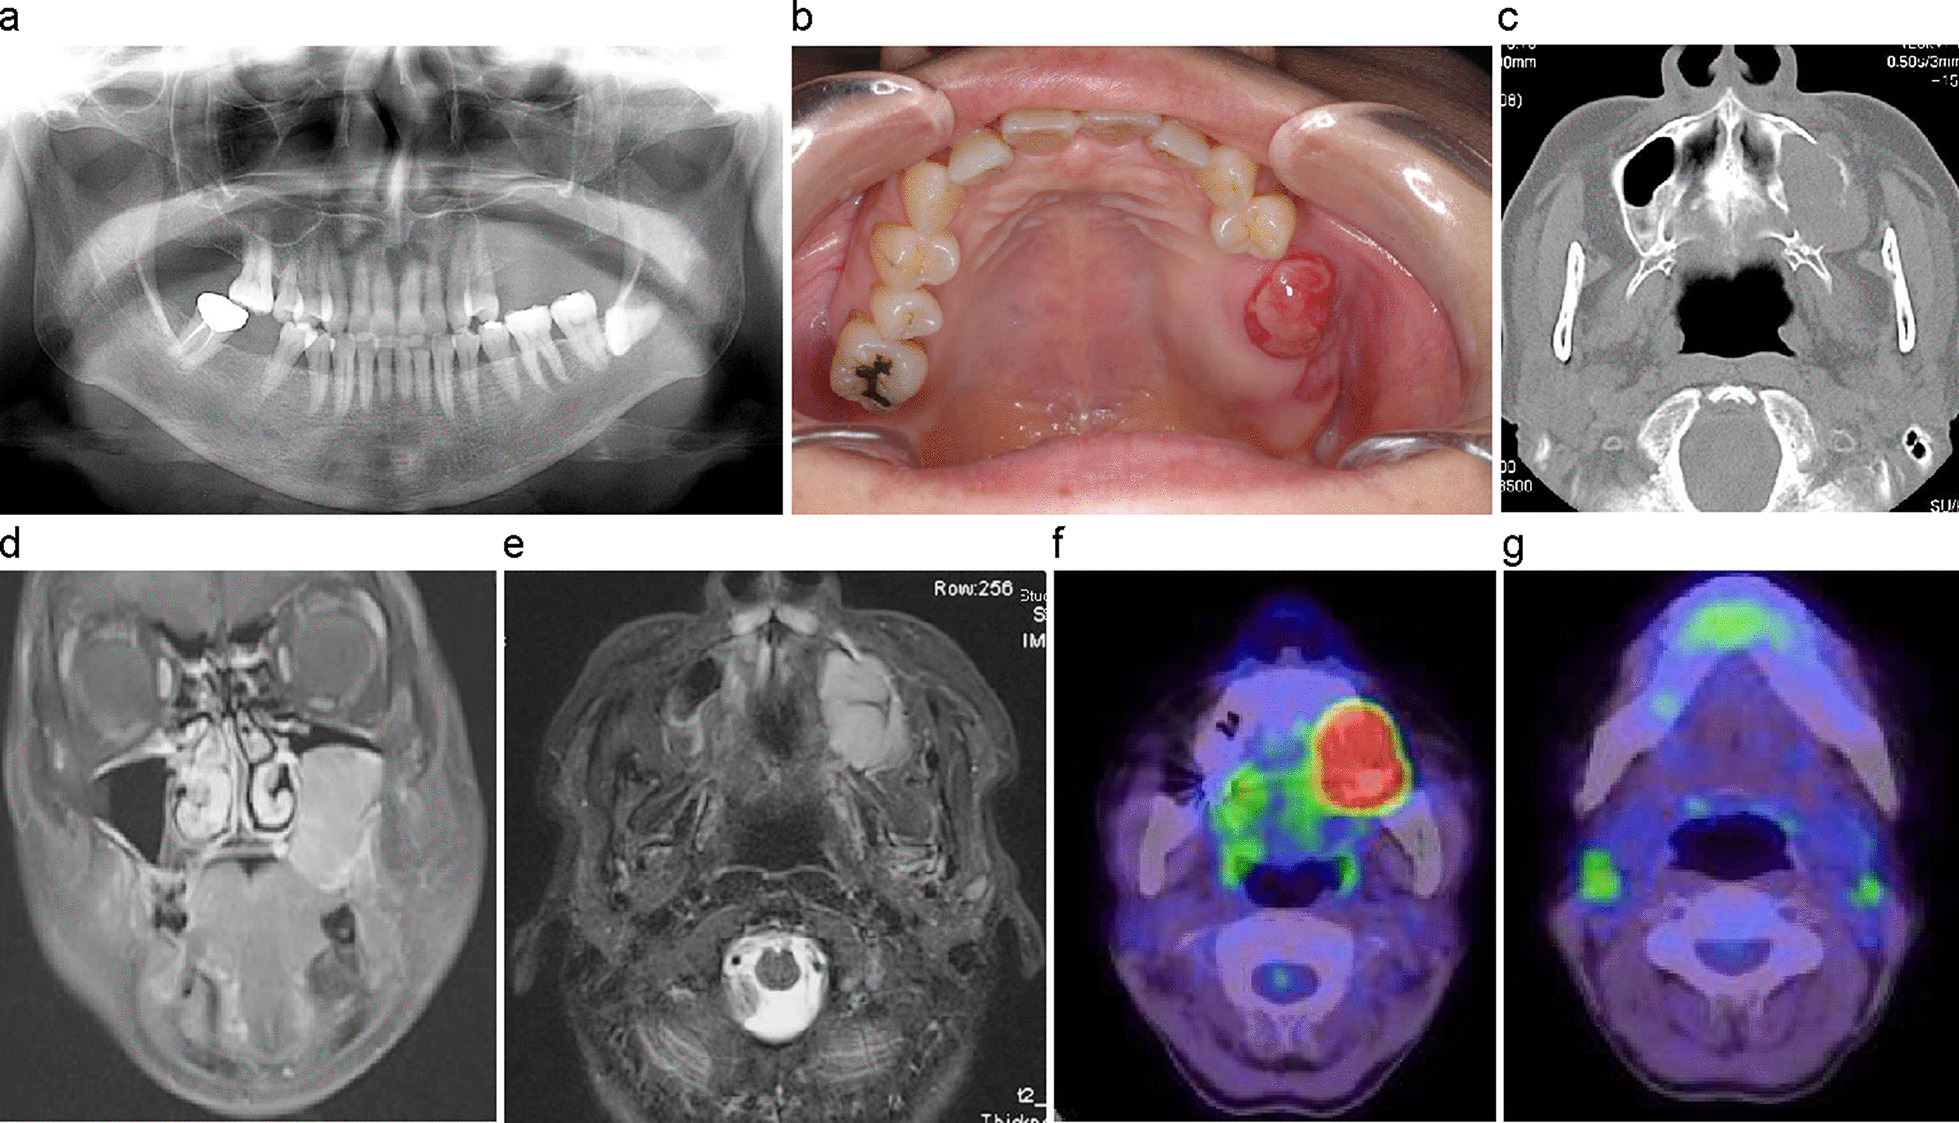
Case 2. A 59-year-old female with DLBCL, stage II_E_A. a Panoramic radiograph image taken at the initial visit showing bone loss at the base of the maxillary left sinus. b Intraoral view of the maxilla, initial visit. c Contrast-enhanced CT showing a neoplastic lesion located in the maxillary bone in the maxillary left sinus region with permeable resorption of the surrounding bone. d Contrast-enhanced MRI showing a mass with low signal on T1-weighted images. e Contrast-enhanced MRI showing high-signal mass on T2-weighted images. f Fluorodeoxyglucose PET/CT showing a lesion with SUV_max_ of 33.2. g Fluorodeoxyglucose accumulation of up to 3.7 in the right cervical lymph node
